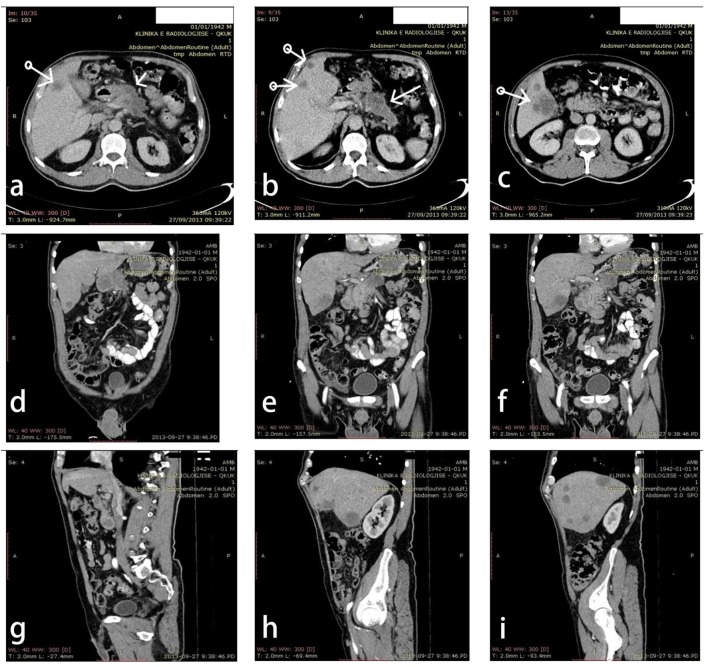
Multidetector computed tomography: axial plane (a, b, c); coronal plane (d, e, f); sagittal plane (g, h, i); results are highly suggestive of primary neoplastic process on the body of the pancreas with multiple liver metastasis and lymphadenopathy along hepatoduodenal ligament, and retropancreatic and intraperitoneal regions.
